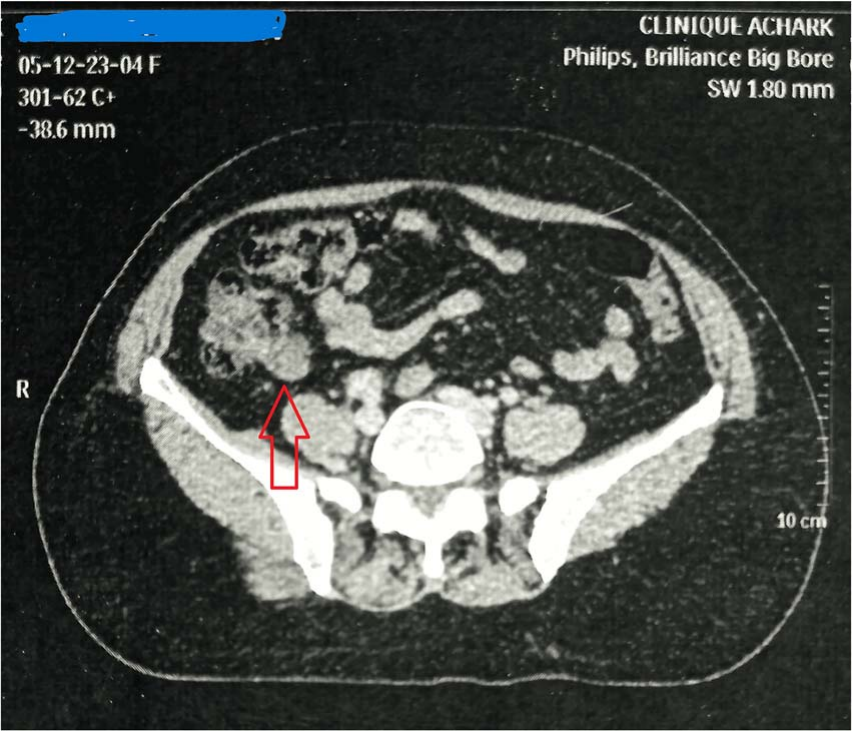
CT image showing an appendicular solido-cystic formation.
